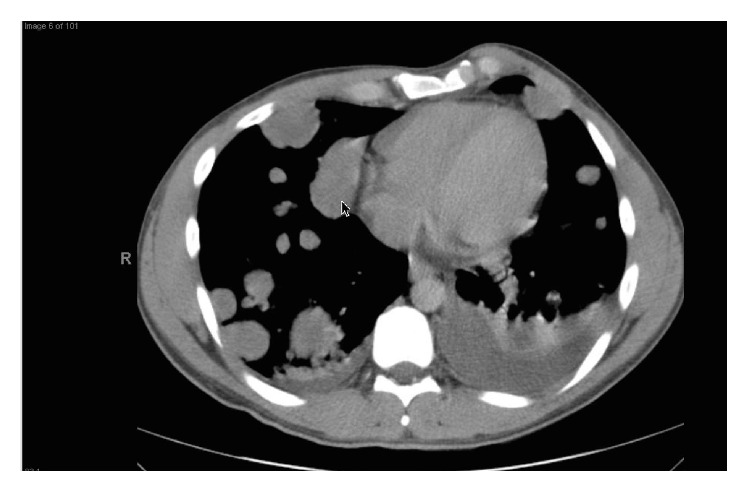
CT of the chest, revealing extensive metastatic disease with numerous pulmonary nodular masses and bilateral pleural effusions.
